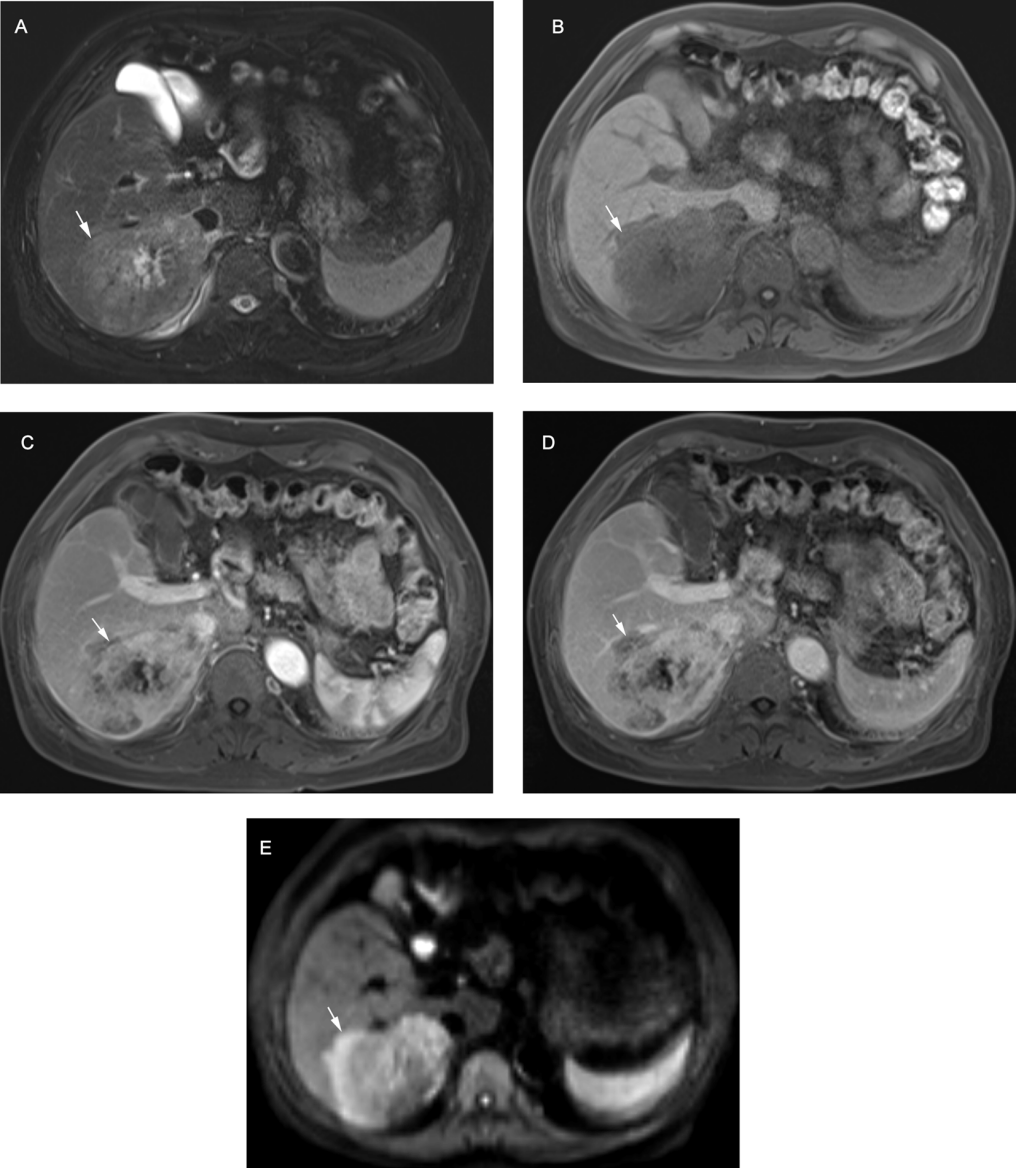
Intrahepatic cholangiocarcinoma in a 74-year-old man (white arrows). (A) T2-weighted image shows a mild hyperintense tumor with central necrosis. (B) T1-weighted image shows a hypointense tumor. Contrast-enhanced (C) arterial phase image shows non-rim hyperenhancement; (D) delayed image shows a non-washout (persistent) enhancement pattern. (E) Diffusion-weighted image (b = 500 s/mm^2^) shows targetoid restriction. The lesion is categorized as LR-M
